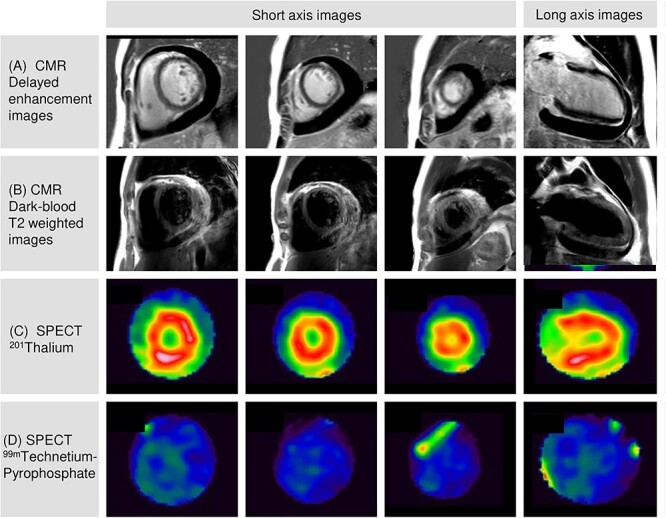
CMR and SPECT. CMR revealed no findings of delayed enhancement (A) and T2-weighted high signal (B) in the myocardium and pericardium. ^201^Thalium SPECT demonstrated normal myocardial perfusion (C). ^99m^Technetium-pyrophosphate did not accumulate in the myocardium (D). CMR: cardiac magnetic resonance; SPECT: single-photon emission computed tomography.
